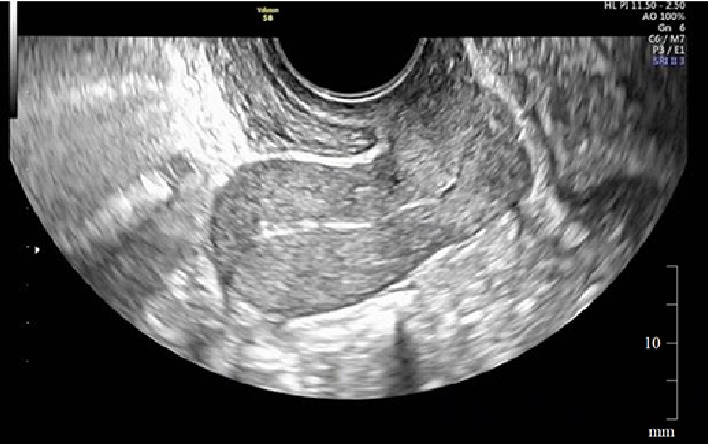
Sagittal ultrasound image of the uterus on the 115^th^ postpartum day. The placenta has become undetectable in the uterine cavity. Note that myometrial thickness has returned to normal.
